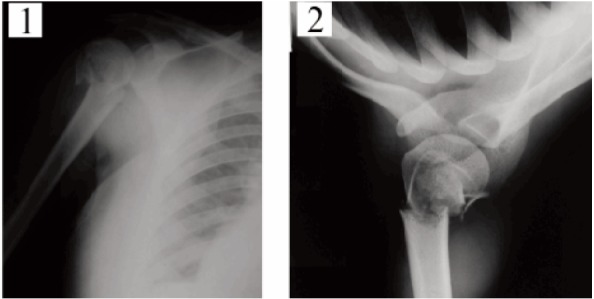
Fig. 2: Posoperative radiograph showing good reduction. F2:
